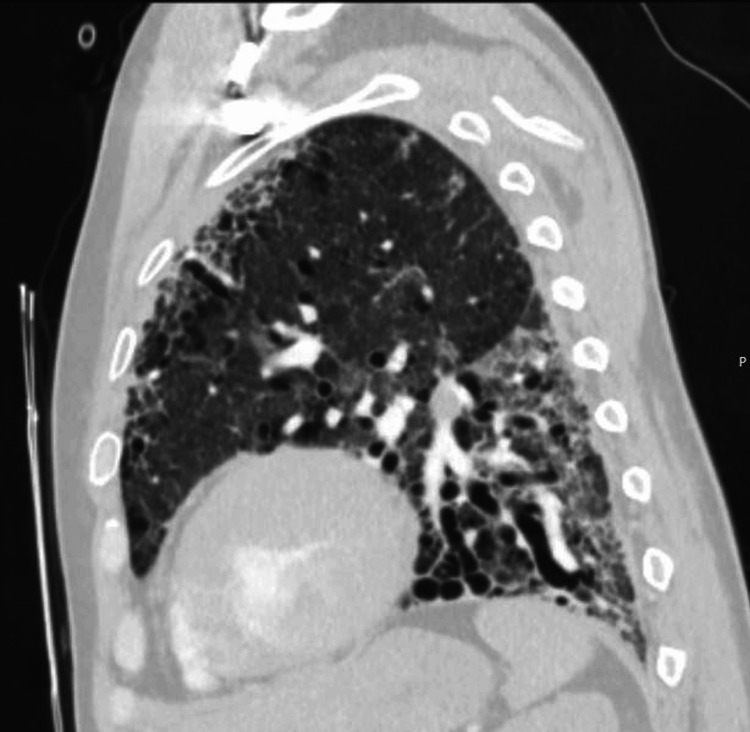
Sagittal chest CT. Sagittal reconstruction highlighting basilar-predominant fibrosis with traction bronchiectasis. No definitive honeycombing is seen. The distribution and imaging features are consistent with a fibrotic non-specific interstitial pneumonia pattern.
